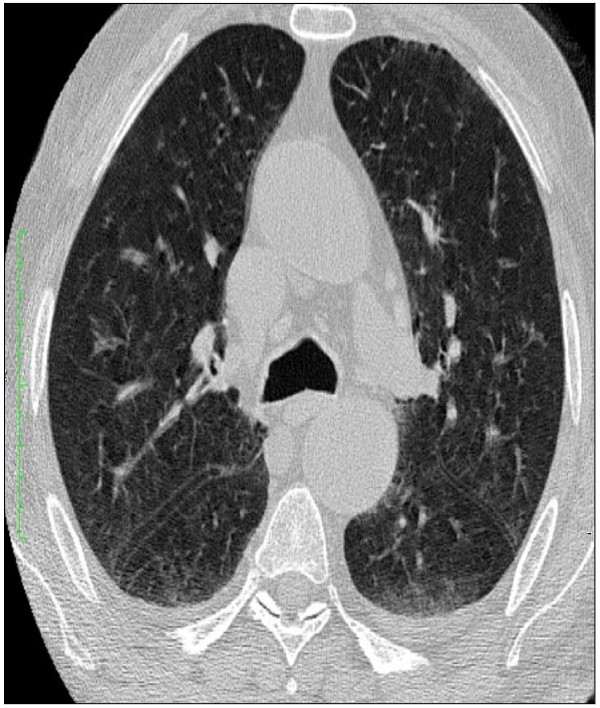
High resolution computed tomography (HRCT) showed minimal emphysematous areas over both apices and non-specific sequalae.
